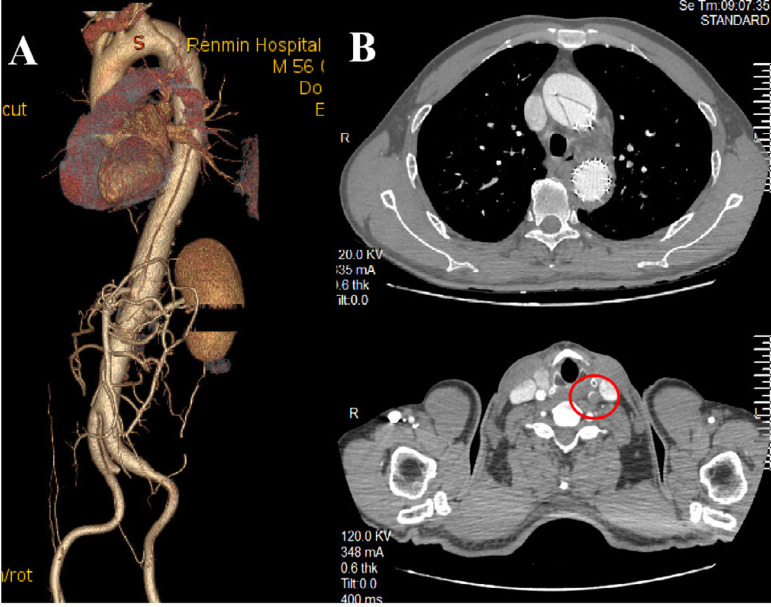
A) Computed tomography angiography of Case 2 showing type B aortic dissection before a thoracic endovascular aortic repair (TEVAR) procedure. B) Shows the retrograde tear after TEVAR forming type A aortic dissection and common carotid artery (CCA) dissection, while the red circle shows a large thrombus in the false lumen of left CCA, leading to a severe narrowing of the true lumen.
